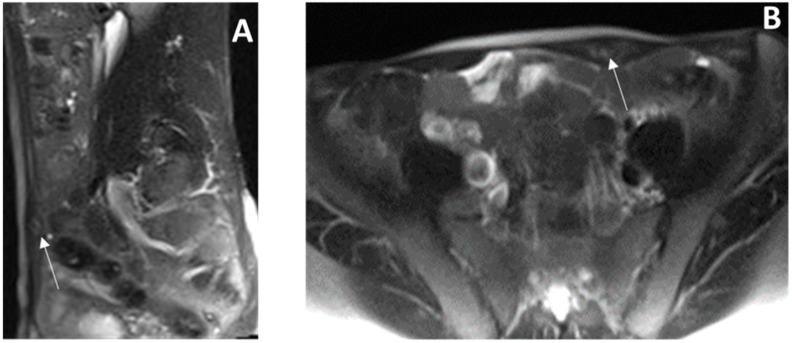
Magnetic resonance imaging (MRI) of abdomen and pelvis. Sagittal (A) and axial (B) HASTE sequence on magnetic resonance imaging (MRI) of abdomen and pelvis (like T2 weighted imaging with fat suppression) reveals enlarged left rectus abdominis muscle with ill-defined hyperintense lesion (arrow).
